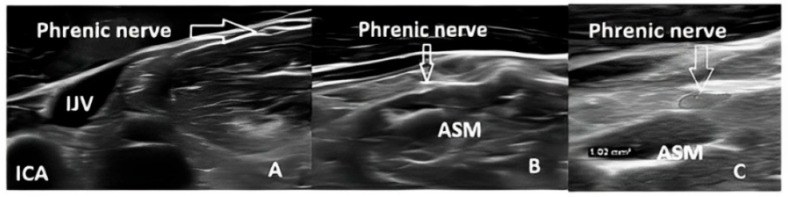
(A) Normal phrenic nerve ultrasound in a healthy volunteer. (B) Phrenic nerve ultrasound in a participant with ALS. (C) Methodology for measuring the cross-sectional area of the phrenic nerve. ASM—anterior scalene muscle; IJV—internal jugular vein; ICA—internal carotid artery.
